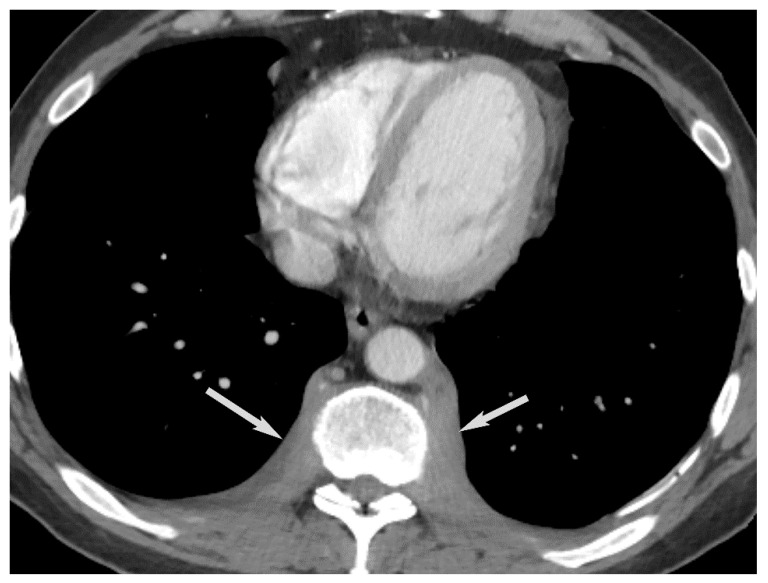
Extramedullary hematopoiesis. CT shows bilateral paraspinal soft tissue thickening (arrows) in a patient with leukemia and anemia.
